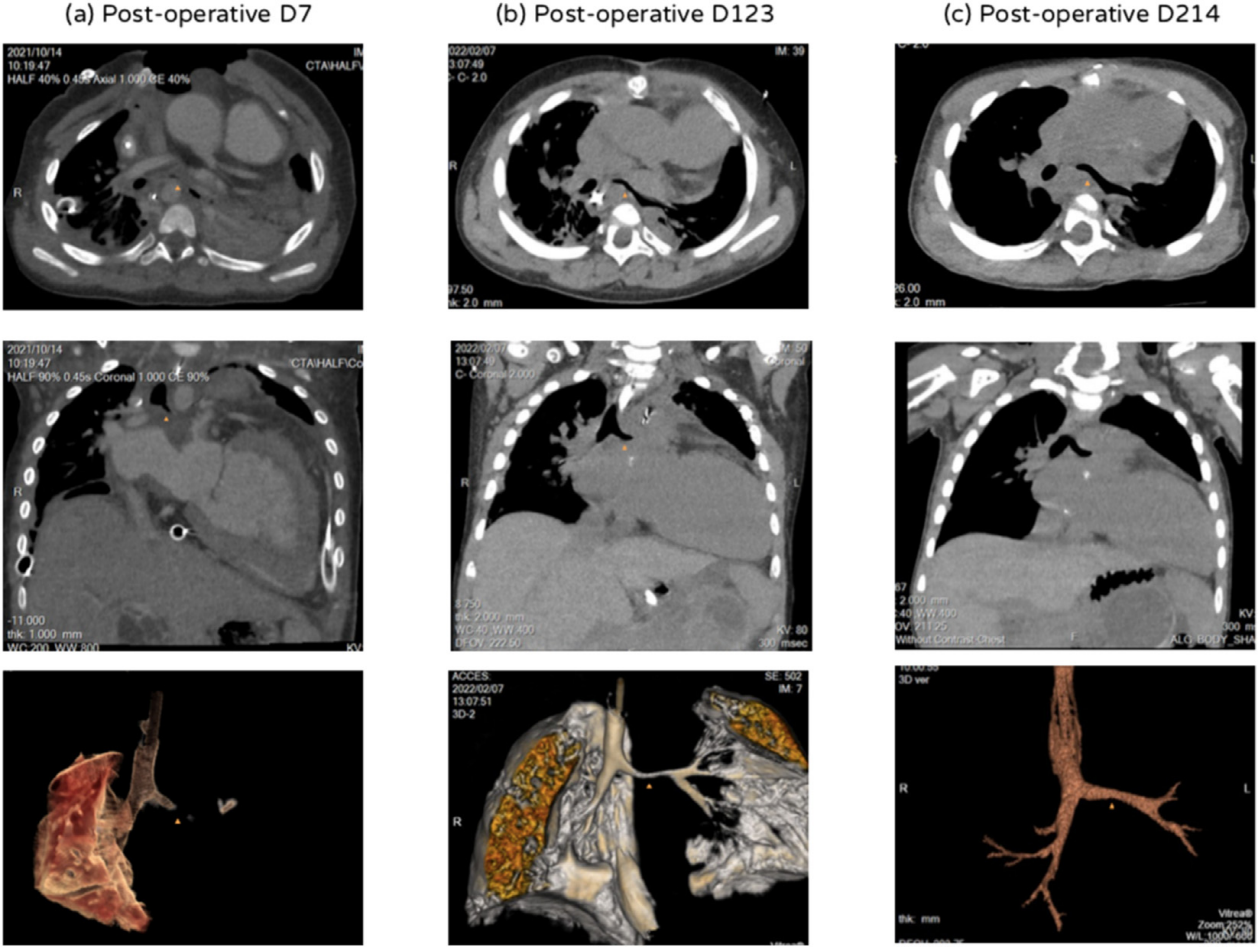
Computed tomography (CT) with image reconstruction (a, left panel) CT on POD 7 showed that the heart deviated the tracheobronchial tree to the right (upper), compressed left bronchus (lower), and occupied almost the entire left hemithorax (middle) (b, middle panel) CT on POD 123 showed a decrease in the size of the cardiac allograft (upper), relieved compression of the left bronchus (middle) and improved expansion of left lung (lower) (c, right panel) CT on POD 214 showed further size remodeling of the cardiac allograft (upper and middle) and only mild residual compression of the left bronchus (lower). ^▲^left bronchus. CT, computed tomography; POD, postoperative day.
